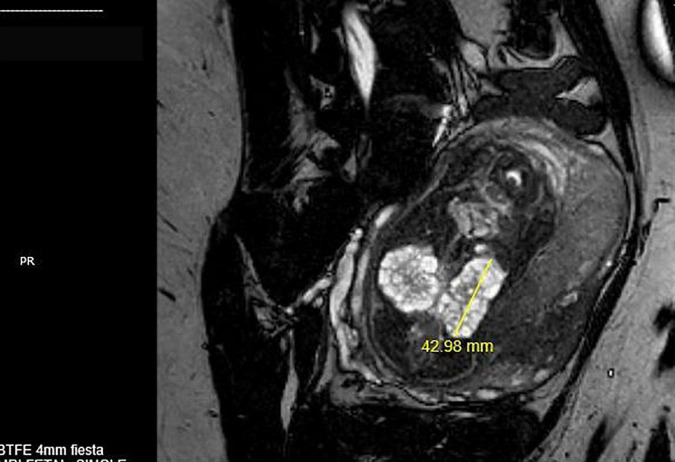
MRI of a singleton fetus in utero at 25 weeks of gestation with bilateral multicystic dysplastic kidneys and absence of amniotic fluid.
